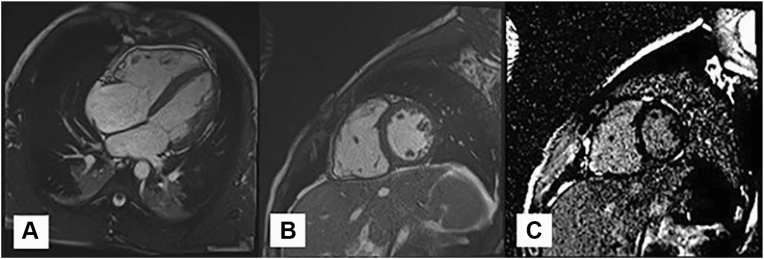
Cardiac MRI on hospital day 4 demonstrating (A) enlarged RV with systolic antero-inferior regional wall dyskinesia in 4-chamber gradient echo sequence; (B) diastolic wall motion abnormality in short axis gradient echo sequence; and (C) late gadolinium enhancement in area of wall motion abnormality on phase-sensitive inversion recovery (PSIR) sequence. MRI = magnetic resonance imaging; RV = right ventricular.
